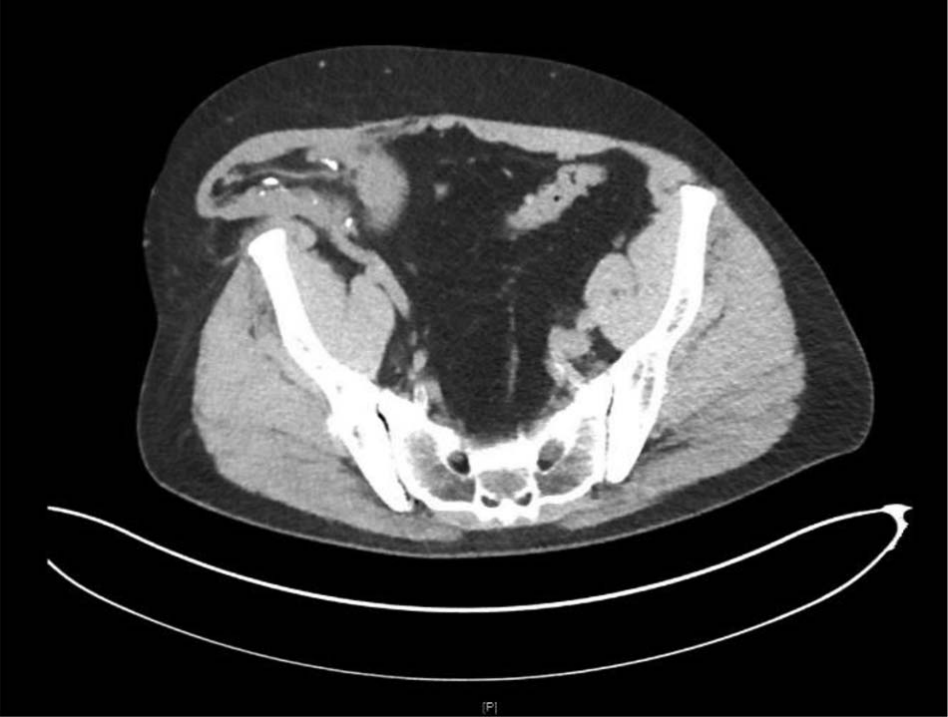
Axial view CT: herniation of the transplanted kidney through the right abdominal wall.
